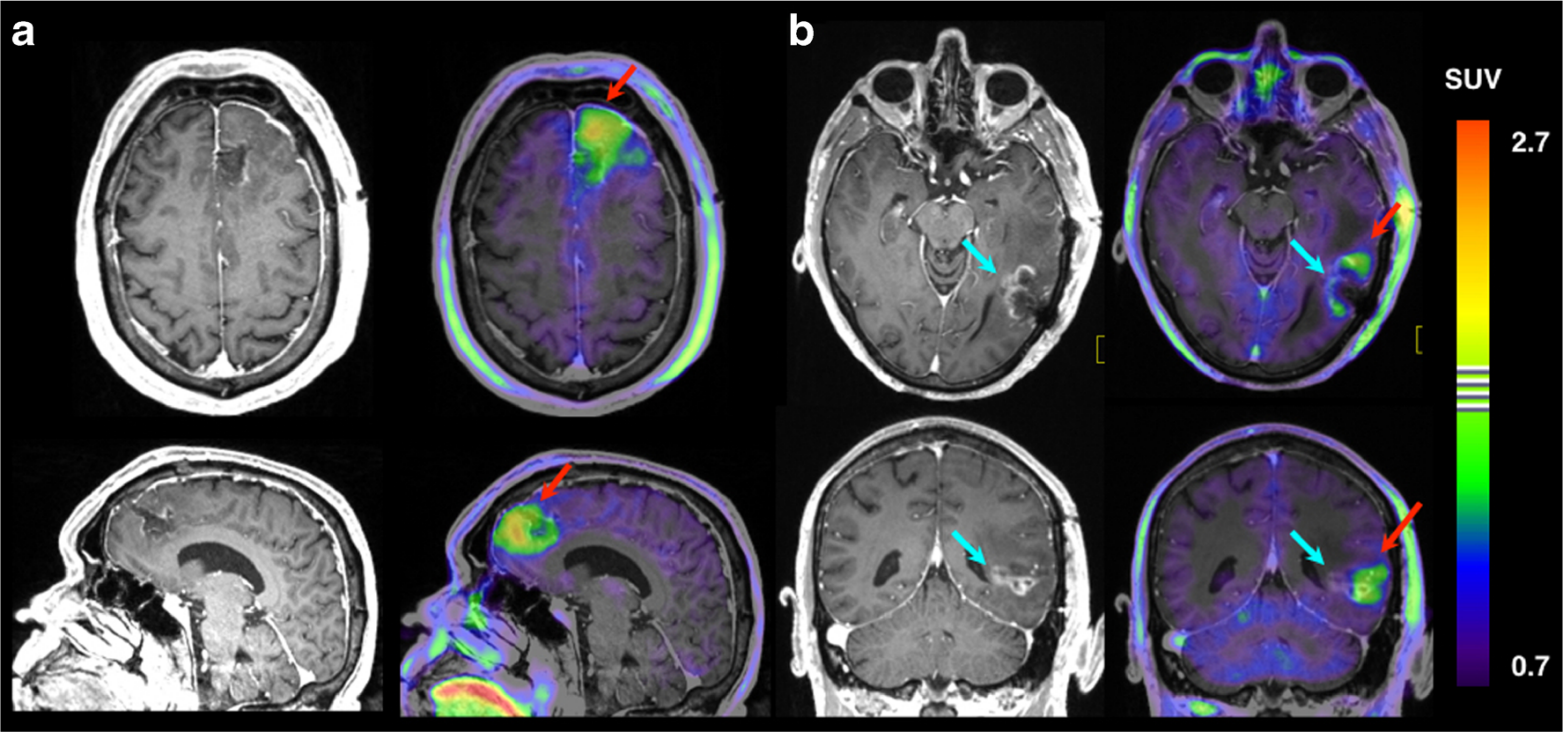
FET PET and early postoperative T1-weighted MRI. Early (48–72 h) postoperative post-contrast T1-weighted MRI alone and fused to FET PET scanning performed 2 weeks later for radiation treatment planning showing examples of conflicting tumor definitions of the two techniques. a. Residual metabolically active non-enhancing tumor remnant anterior to the resection cavity identified in the left frontal lobe (*red arrow*), presumably infiltrating glioblastoma. On MRI, the tumor was evaluated as gross *total* resected. Patient ID 30. Gd + MRIvol: 0 cm^3^; BTV: 26 cm^3^; OS: 13 months. See Table S1. *Top*: Transaxial section above corpus callosum. *Bottom*: Sagittal section left of midline. This patient was evaluated as *partially* resected based on a smaller contrast enhancing region (*blue arrow*) in the depth of the resection cavity in the left temporal region. Lack of metabolic activity on FET PET, however, suggests reactive changes. Areas of increased uptake is found in subcortical white matter anterior and posterior to the cavity (*red arrow*), presumably infiltrating glioblastoma. Patient ID 98. Gd + MRIvol: 3.2 cm^3^; BTV: 7.8 cm^3^; alive at 31 months follow-up. See Table S1. *Top*: Transaxial section at the level of mesencephalon. *Bottom*: Mid-cerebellar coronal section
